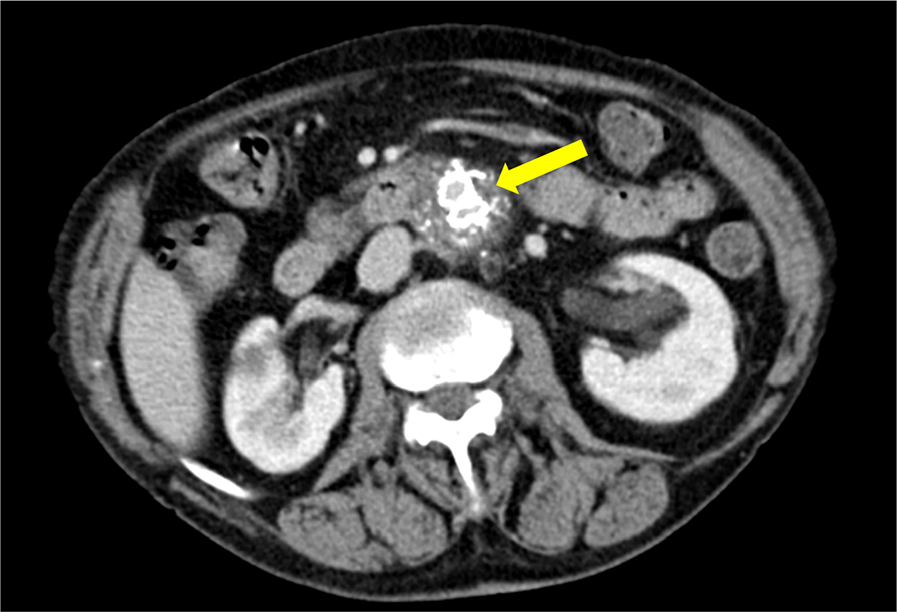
CT-angiography control at 6 months. The arrow indicates the slight narrowing of the iliac legs of the graft.
